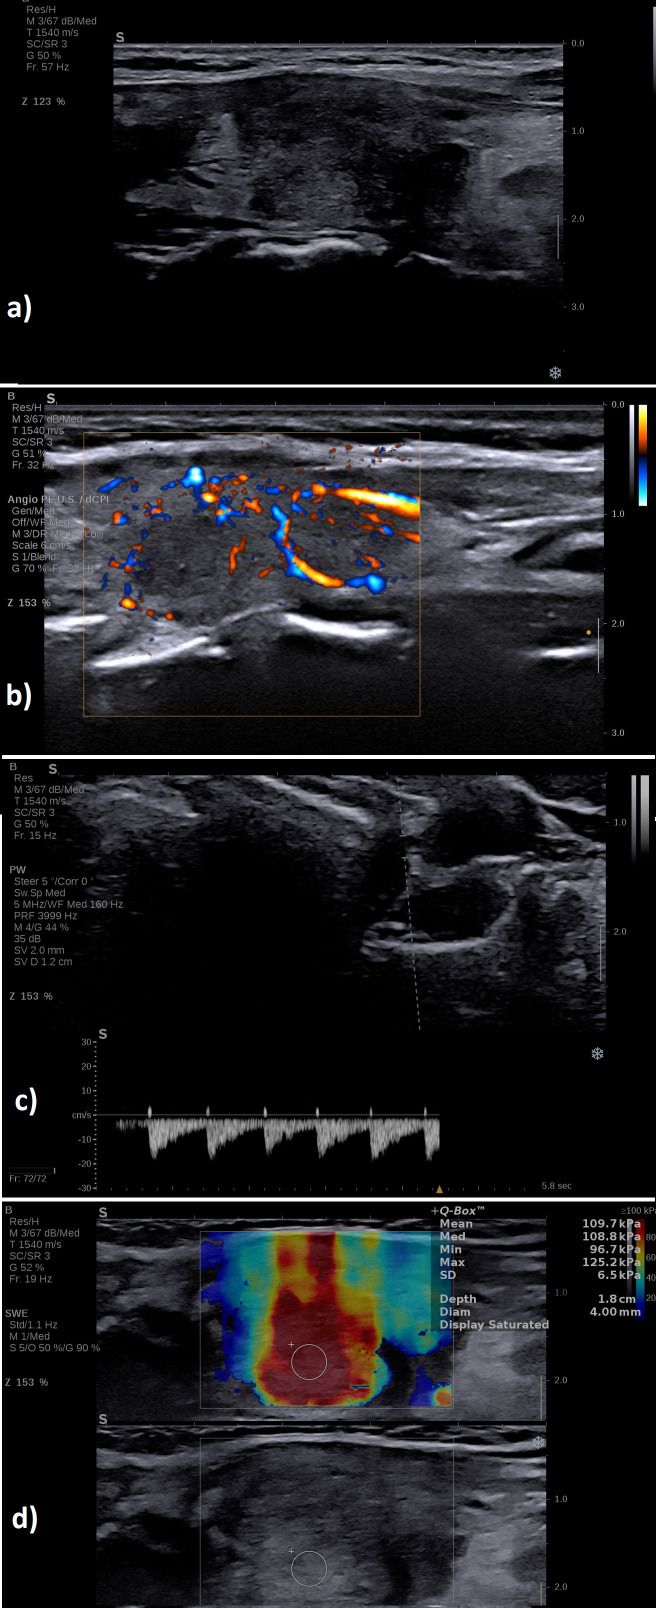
A case of a patient with Graves’ disease: (A) B-mode examination of the entire thyroid in transverse plane: diffusely enlarged with enlarged isthmus of 9 mm, diffusely hypoechoic; (B) with increased vascularity grade III Color Doppler, image is obtained using the fine-flow mode called ANGIOPLUS on the equipment used in this study; (C) spectral Doppler increased PSV in the thyroid artery; (D) normal mean elasticity index measured in 2D SWE 12 kPa.
